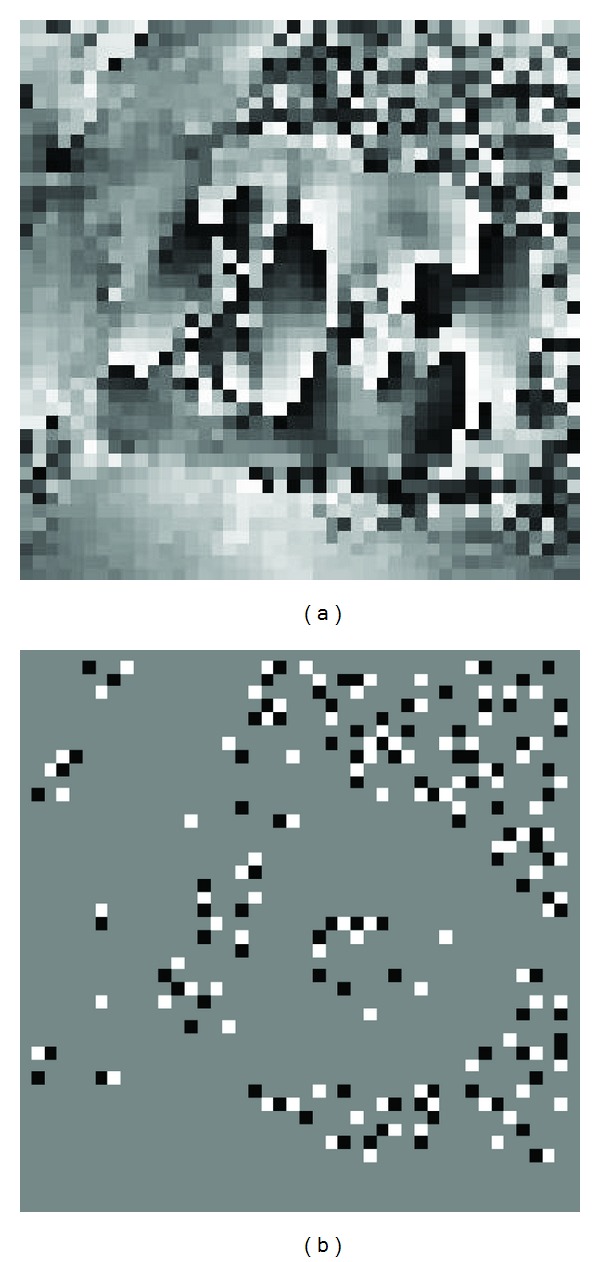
(a) A 44 × 44 displacement encoded MRI heart phase image, (b) its corresponding residue distribution involving 396 residues, 198 positive polarity residues, and 198 negative polarity residues.
